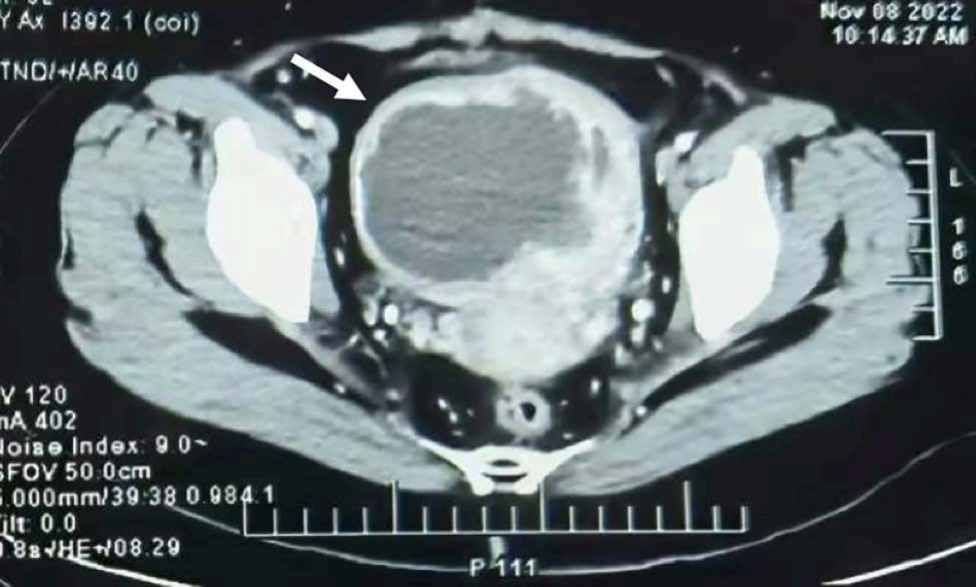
Enhanced CT scans showed an abnormal mass in the uterus. CT = computed tomography.
